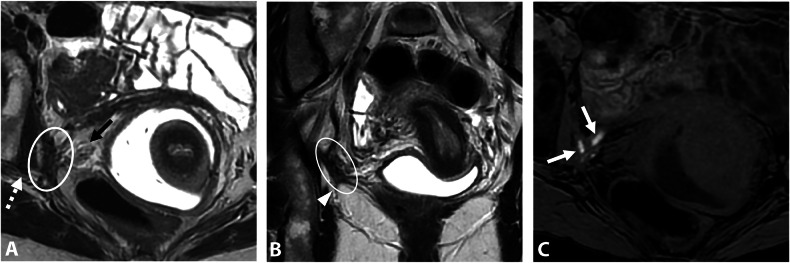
DE in a 43-year-old woman with dyspareunia and perineal pain. A Axial and (B) coronal T2W MR images show an endometriotic lesion (circle) of the right distal mediolateral parametrium extending to the pelvic wall in contact with the pudendal vascular-nerve bundle at the level of arcus tendineus of the levator ani muscle (B, arrowhead). The right ureter passes quite widely inside this lesion (A, arrow). This lesion is located in front of the right ischial spine mark (A, dashed arrow) and the sciatic nerve. This lesion is isolated without involvement of the ovarian fossa or the posterolateral parametrium. C Axial fat-suppressed T1W MR image showing diffuse T1-hyperintense endometriotic hemorrhagic microcysts (arrows). The patient was treated medically with hormone therapy
